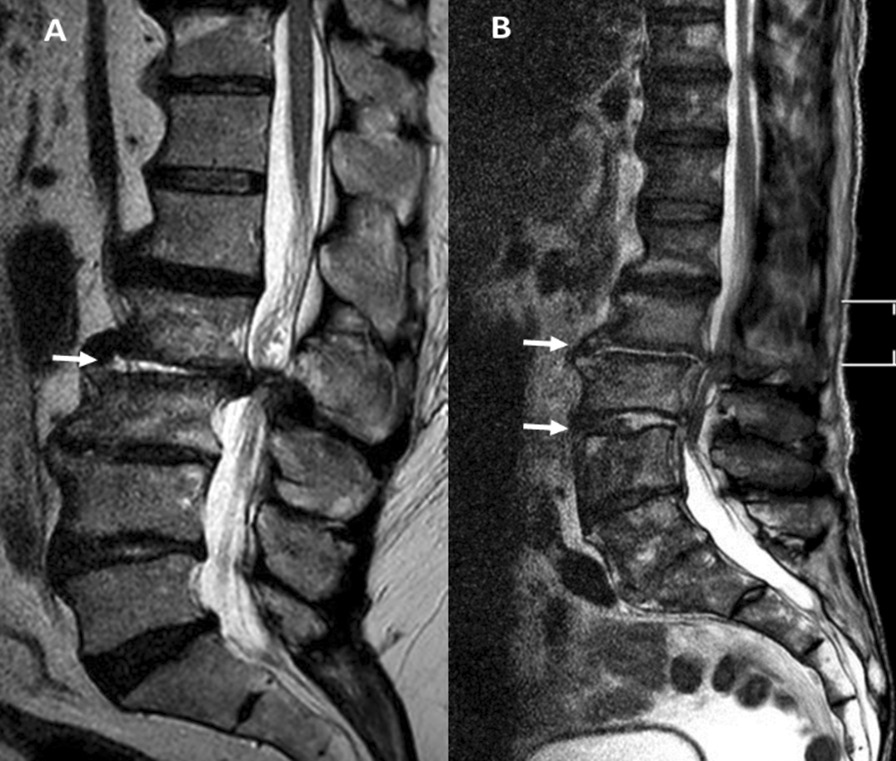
MRI STIR sagittal sequence: SL PSD (A) and adjacent ML PSD (B)
